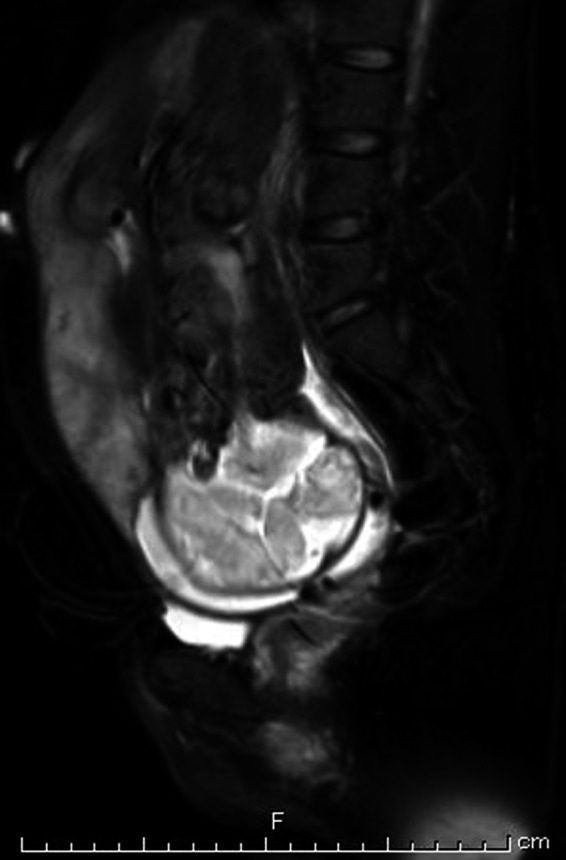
T2‐weighted MRI confirmed the normal placental position and the absence of abnormal placental morphology
